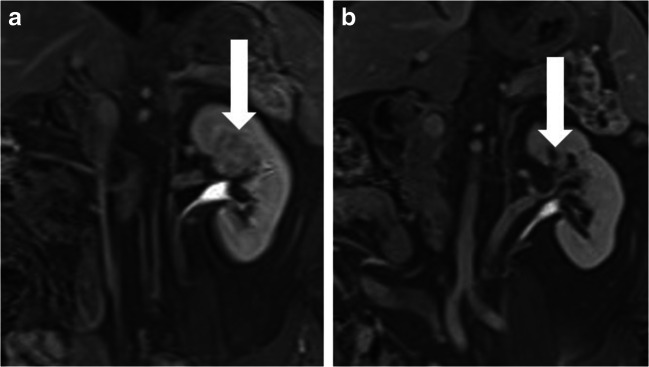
Coronal 12-min excretory phase post contrast-enhanced MRI showed a centrally located 3.5-cm biopsy-proven grade 1 eosinophilic RCC (vertical arrowed) pre-IRE (a) and complete resolution of tumour (vertical arrow) at 12 months post-IRE (b)
